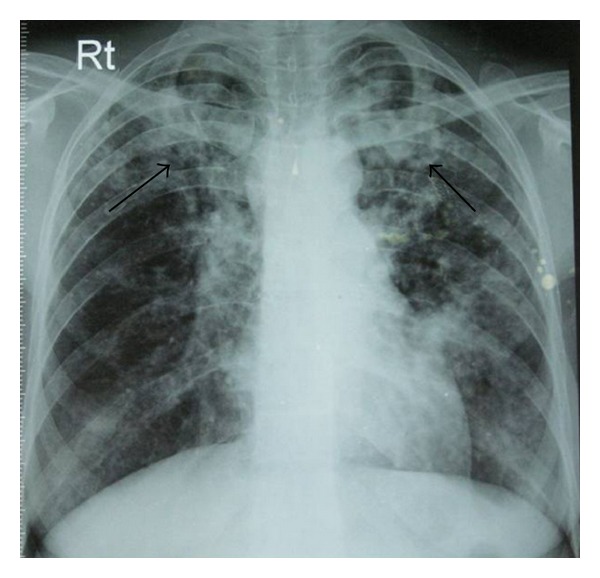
Initial chest radiograph showing bilateral infiltrates (arrows).
